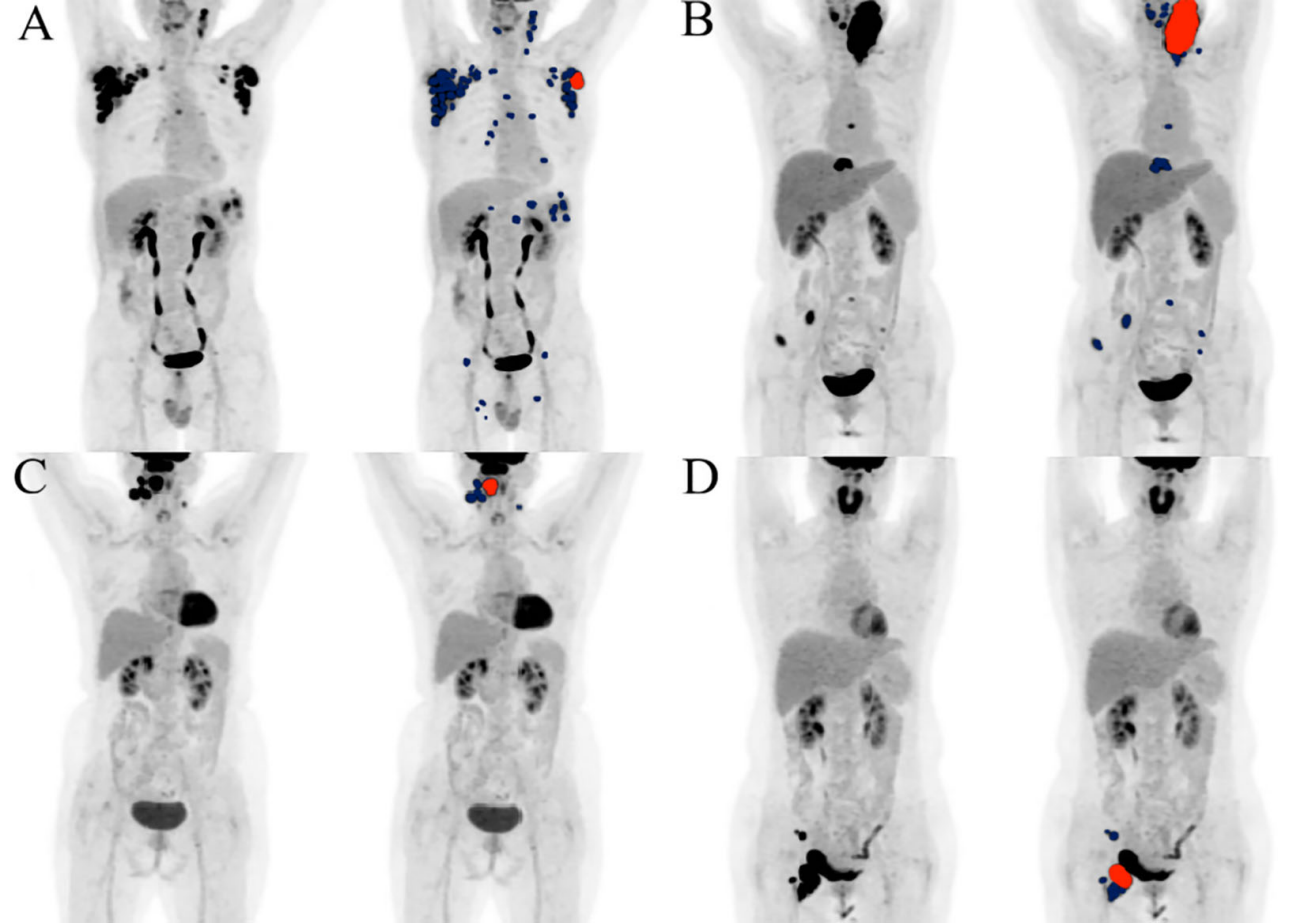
TMTV and Dmax from ^18^F-FDG PET/CT assess tumor burden and dissemination, respectively. MBV reclassified risk in two representative patients (A, B) with both high TMTV and high Dmax, as well as in another two (C, D) with both low TMTV and low Dmax. All involved lesions are indicated in blue, and the largest lesion used to derive MBV is highlighted in red. Patient (A) had high TMTV (99.71 cm³), high Dmax (68.8 cm), and low MBV (11.74 cm²), remaining progression-free and alive after 29 months of follow-up. Patient (B) showed high TMTV (100.23 cm³), high Dmax (59.24 cm), and high MBV (82.89 cm²); progression occurred at 3 months and death at 7 months of follow-up. Patient (C) had low TMTV (21.04 cm³), low Dmax (9.73 cm), and low MBV (6.30 cm²), remaining progression-free and alive after 83 months of follow-up. Patient (D) showed low TMTV (33.03 cm³), low Dmax (10.20 cm), and high MBV (23.78 cm²), with death occurring at 10 months of follow-up.
